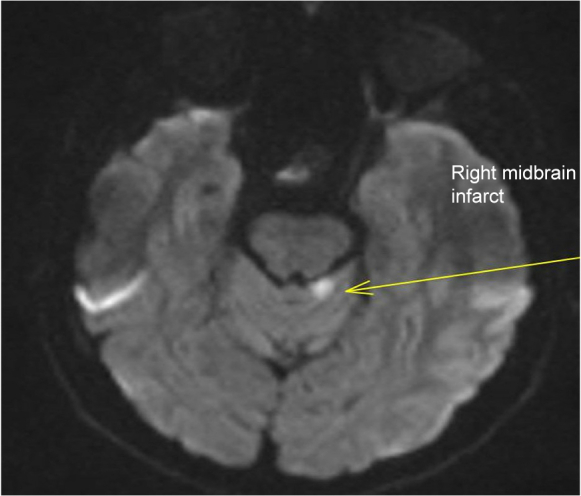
MRI brain noncontrast scan, axial T1 view: multifocal infarcts of the left superior cerebellum, right midbrain, and bilateral ventral medial thalami.
